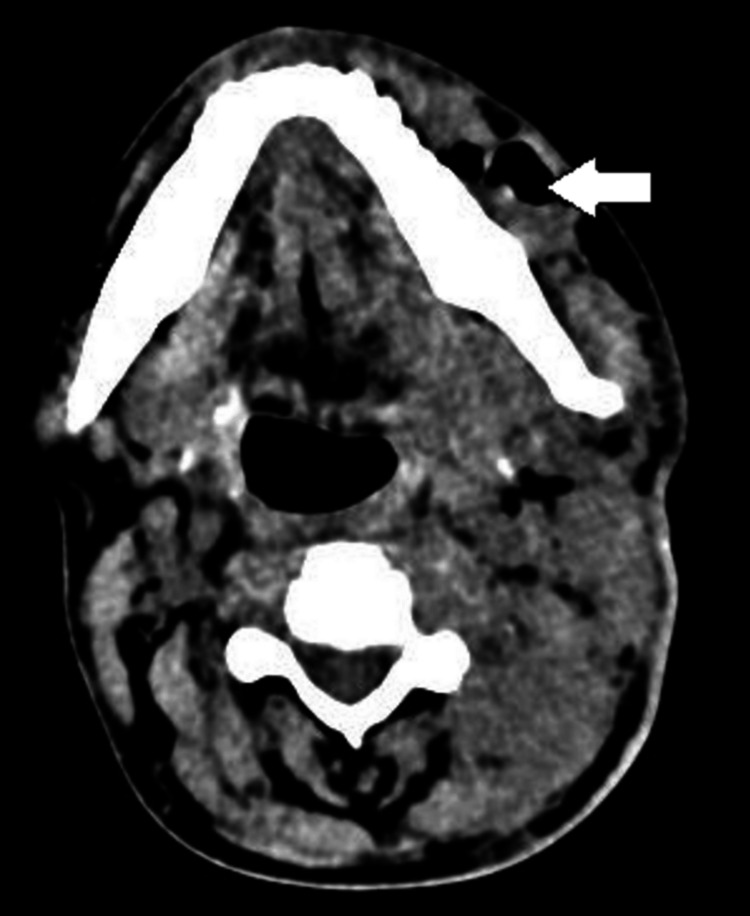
An axial section of computed tomography of the mandible, representing a radiolucent lesion over the left side
